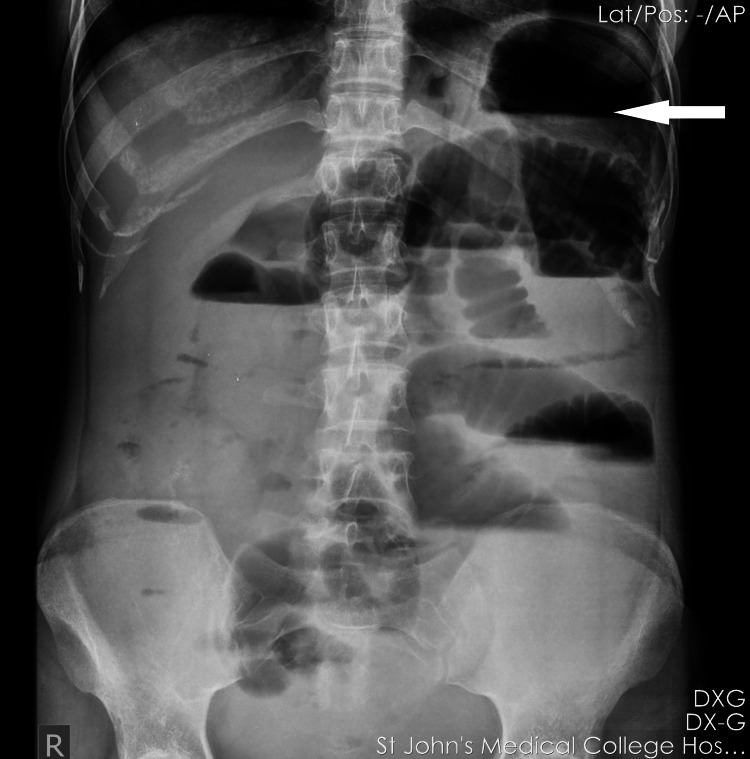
Erect X-ray showing air under the diaphragm, suggestive of gastrointestinal perforation.
